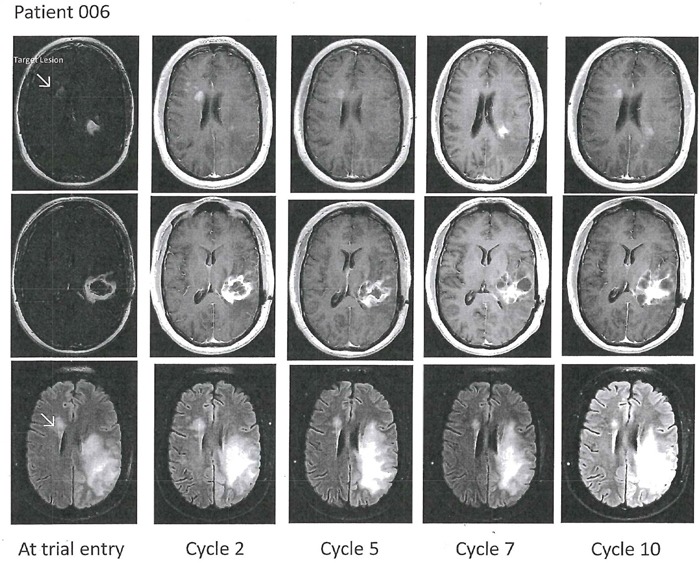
Axial T1-weighted contrast enhanced (top two rows) and T2-FLAIR images (bottom row) in Patient 6 with an original left temporal glioblastoma as depicted in the middle row The patient developed a right frontal periventricular tumor progression, marked with the arrow as the index tumor at baseline. Follow-up images showed stable disease of the index tumor and as for the left temporal tumor component evolving multi-cystic contrast-enhancing changes and edema were noted.
